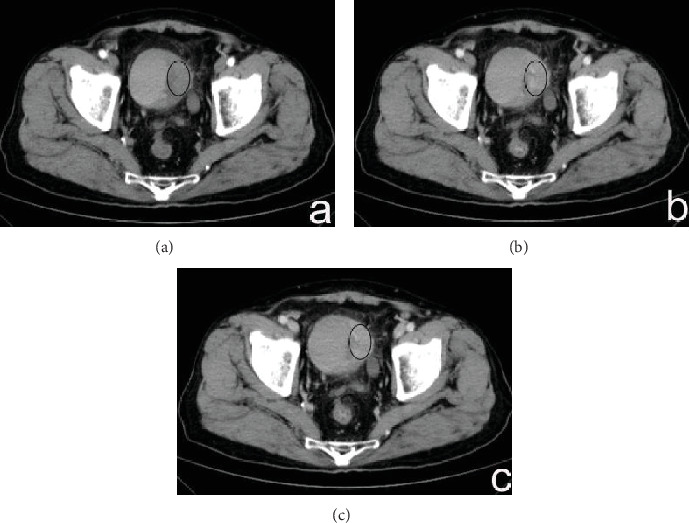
(a–c) A 37-year-old man had CG that presented as mound-like lesions in the left lateral wall of the bladder, including the trigone. The enhanced CT values of the lesions in the arterial, venous, and delayed phases were 43.3, 57.8, and 49.8 HU, respectively. The enhanced CT values in the bladder wall in these three phases were 30.2, 38.9, and 32.5 HU, respectively. The relative enhanced CT values (calculated as enhancement CT values of the lesion − enhancement CT values of normal bladder) in the arterial, venous, and delayed phases were 13.1, 18.9, and 17.3 HU, respectively.
